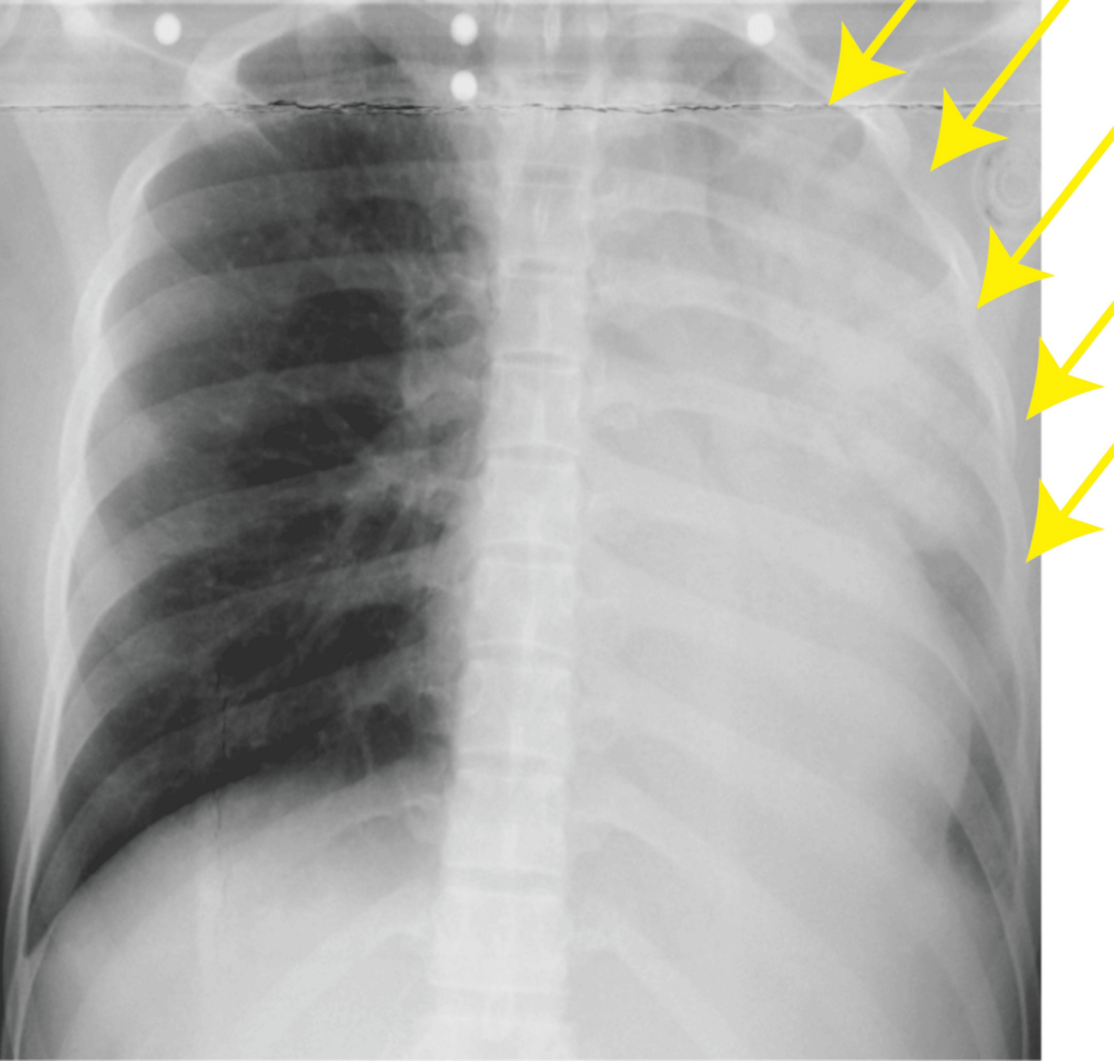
Chest X-ray imaging Postoperative chest X-ray during cesarean delivery. The areas of pulmonary edema are indicated by yellow arrows.
